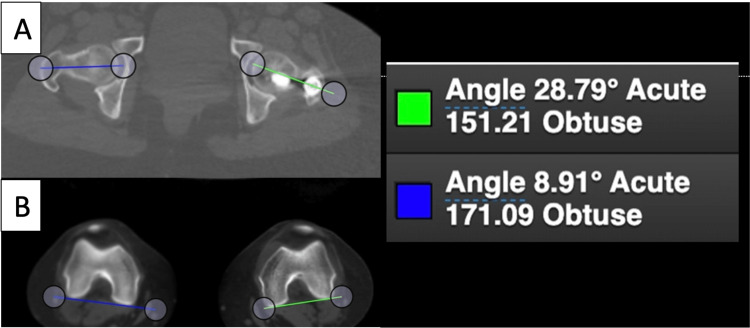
Measurement of femoral version based on CT scanogram axial views. (A) Femoral neck angles bilaterally. (B) Measurement of the posterior condylar axis bilaterally. Legend on right showing the right femur (blue lines) to be anteverted 8.91 degrees and the left femur (green lines) to be anteverted 28.79 degrees. ° = degree
